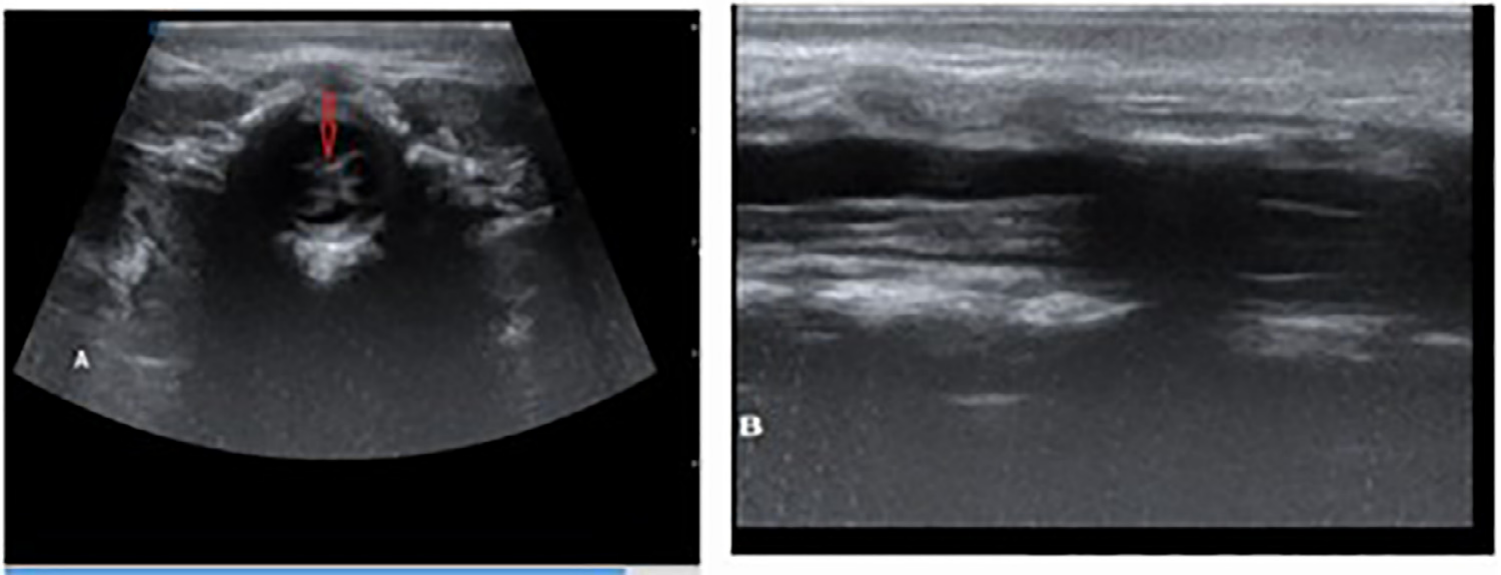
Anteroposterior (AP) radiograph of the thoracic spine showing mild levoscoliosis in the mid-thoracic region. Vertebral anomalies include formation and segmentation defects between T6 and T8 (yellow arrow). The left 5th, 6th, and 7th ribs are completely absent (red star). Partial fusion is noted between the 11th and 12th ribs (yellow star), and the left 9th rib appears bifid (green star). The heart is shifted to the right side (dextroposition) (black arrow).
